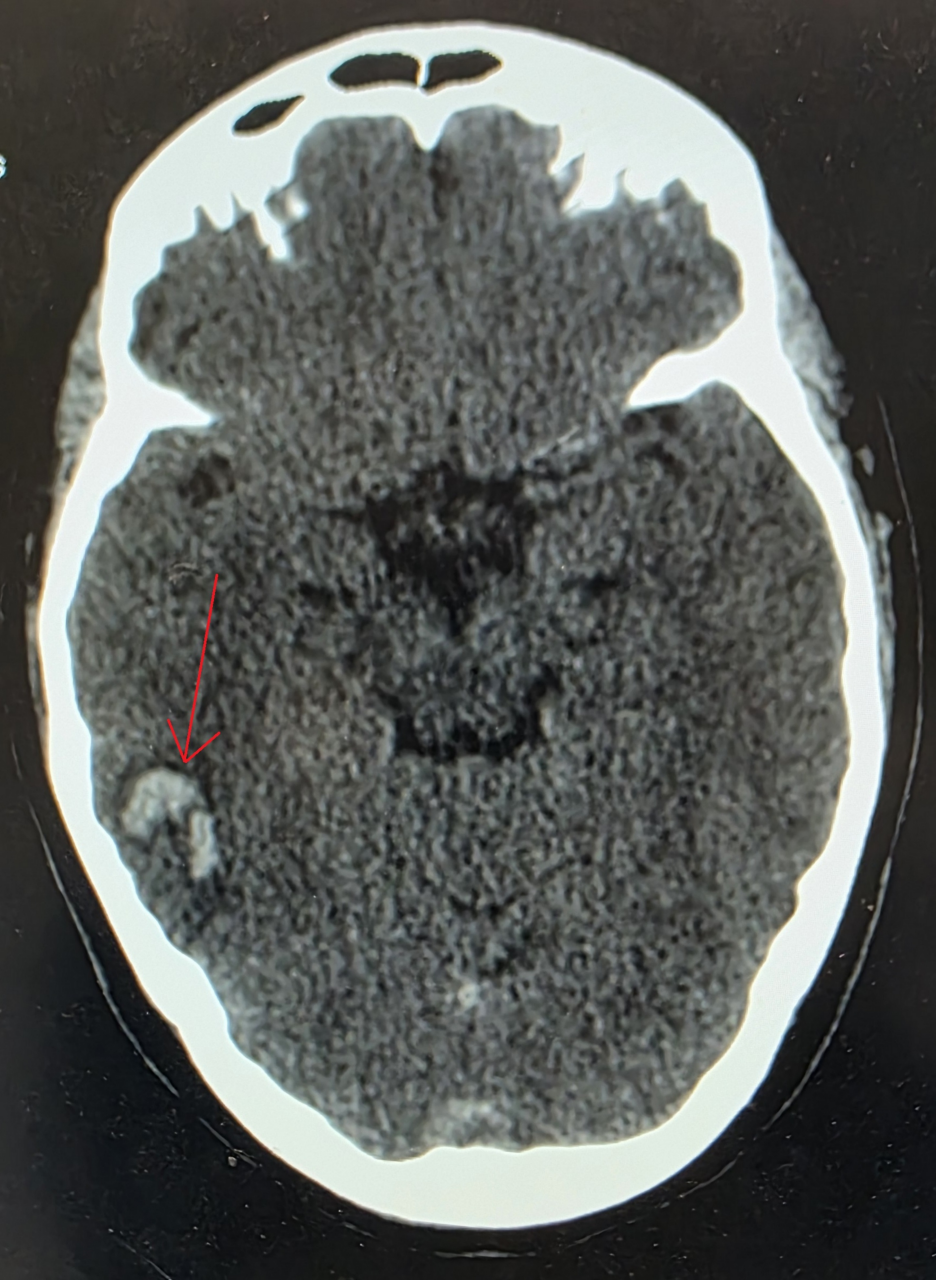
Non-contrast axial brain CT showing a right temporal hemorrhagic infarction (arrow) CT: computed tomography
